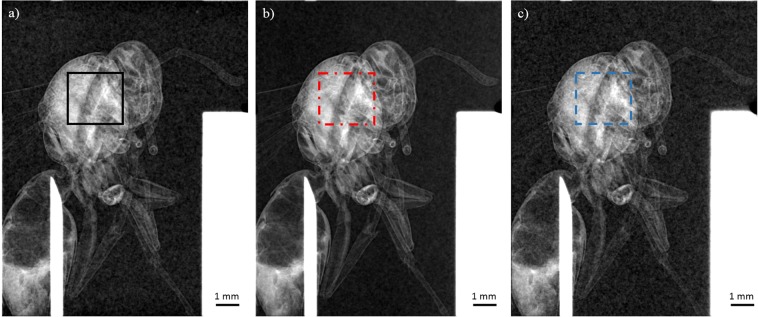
X-ray phase images based on Paganin phase retrieval algorithm^50,51^. (a–c) are retrieved from the raw images presented in Fig. 3 (a–c). (a) for I = 2.7 × 10^17^ W/cm^2^, (b) for I = 1.0 × 10^18^ W/cm^2^, (c) for I = 7.2 × 10^18^ W/cm^2^.
